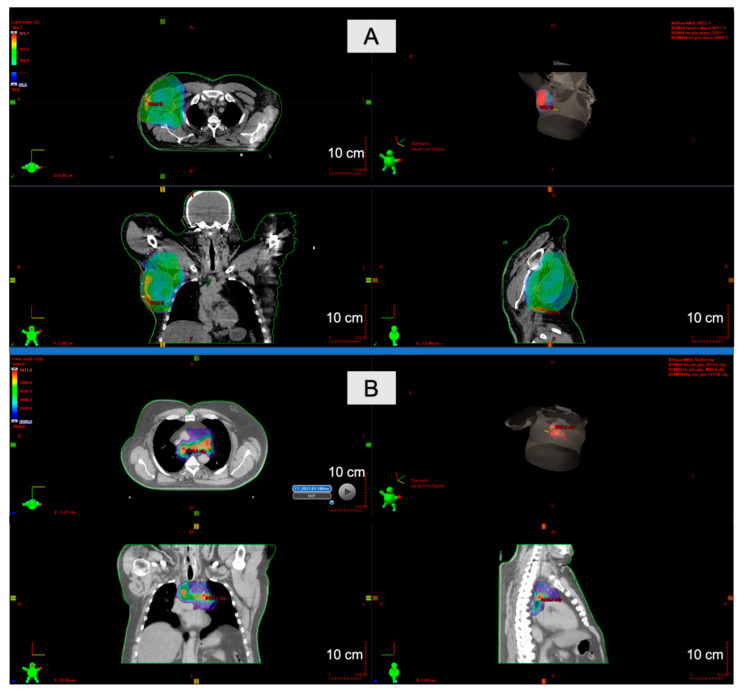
Radiotherapy for eccrine porocarcinoma. The patient received adjuvant radiotherapy (A) after axillary lymph node dissection due to locally advanced porocarcinoma with five lymph node metastases with extracapsular extension using intensity-modulated radiotherapy, 60 Gy in 2 Gy fractions. After six months of follow-up, the patient developed lung metastases, and he underwent chemotherapy followed by pulmonary metastasectomy. The first computed tomography after surgery showed mediastinal lymph nodes metastases. Then, the patient received hypofractionated radiotherapy (B) for oligometastatic disease using volumetric modulated arc therapy with simultaneous integrated boost, 45 and 30 Gy in 4.5 and 3 Gy fractions.
